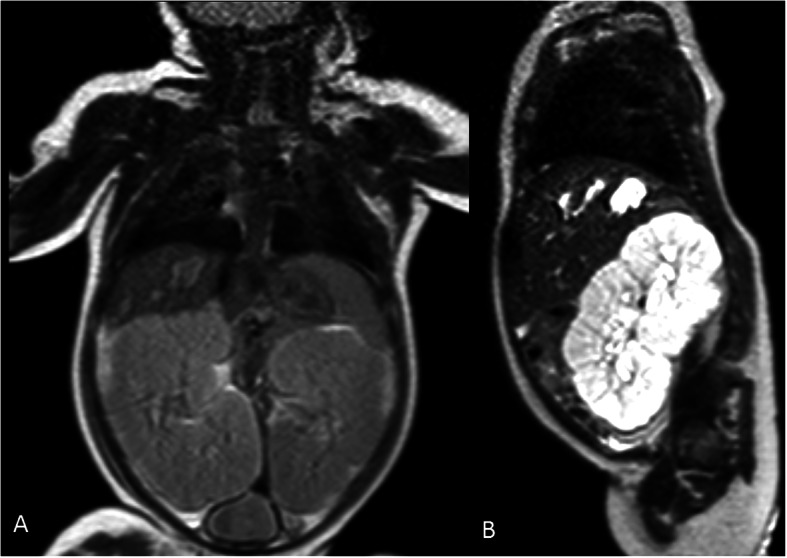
Abdominal magnetic resonance imaging. a. T1 sequence, in coronal plane, shows enlargement of both kidneys (right 8.5 × 5.4 cm, left 8.2 × 5.4 cm), with altered structure and bulged margins, and hepatomegaly with at least two cystic lesions in segments VII and VIII. b. T2 sequence delayed enhanced image, in right parasagittal plane, shows enlarged kidney with inverted structure, and at least two cystic focal lesions in segments VII and VIII of the liver
